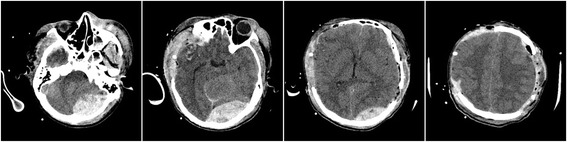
Computed tomography following the second decompressive surgery showing remote delayed epidural hematoma
